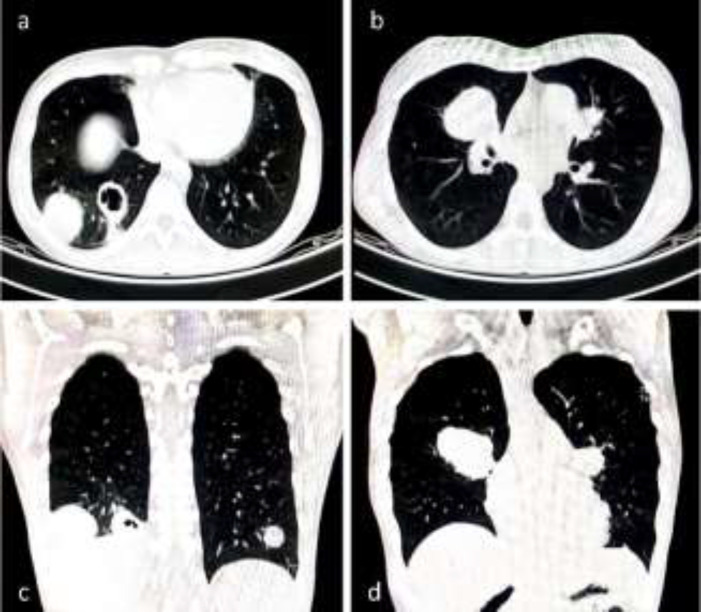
Computed tomography of the chest in axial (a, b) and coronal (c, d) views showed bilateral multiple pulmonary hydatidosis in 28-year-old female patient. The right middle lobe (RML) involvement was more than 70% in this patient. Despite the indication of RML lobectomy, cyst enucleation and broncho-pleural fistula ligation was performed
